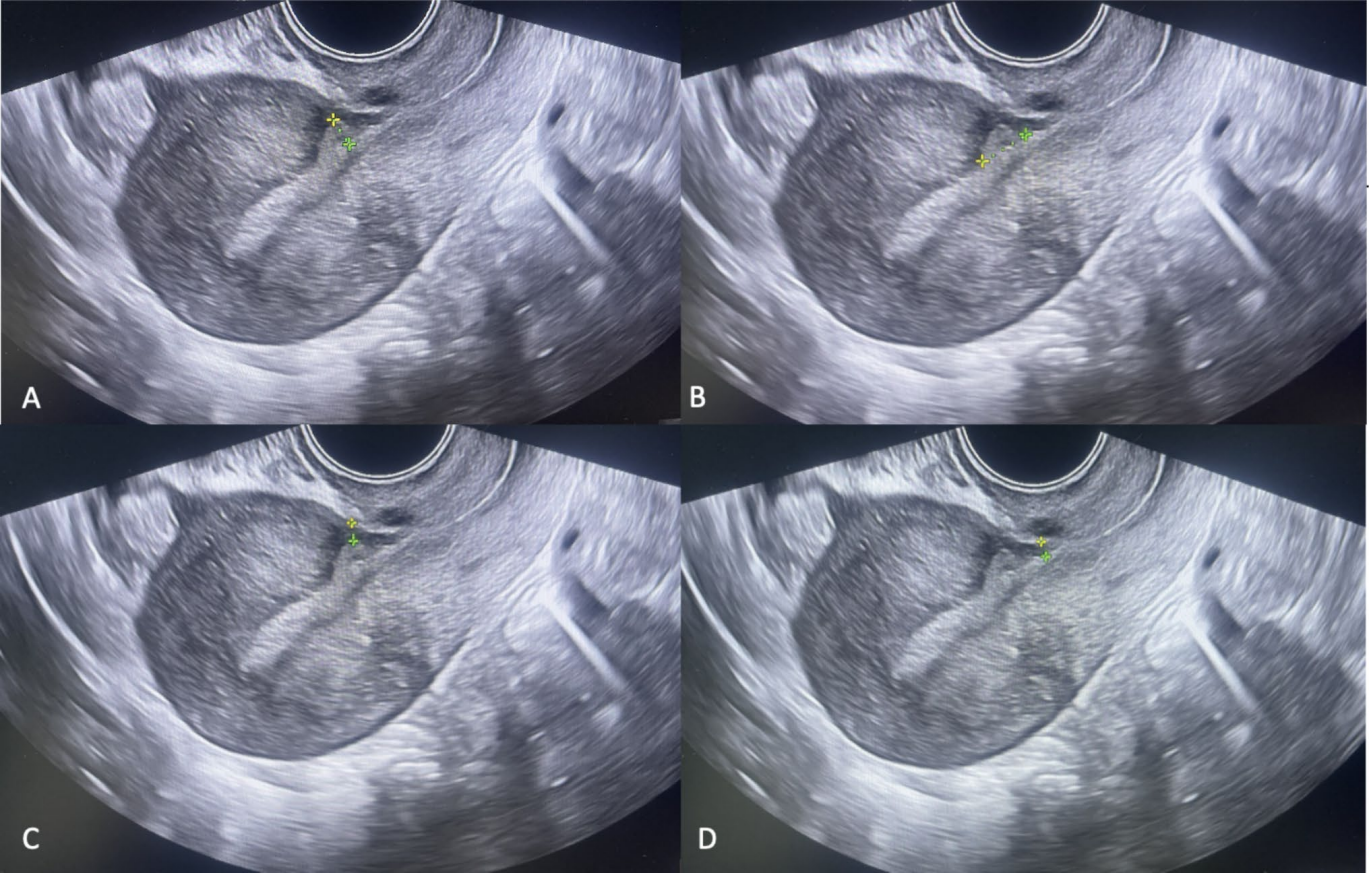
Ultrasonographic representation of isthmocele measurements in the sagittal plane. A Depth; B length; C residual myometrial thickness (RMT); D adjacent myometrial thickness (AMT)
